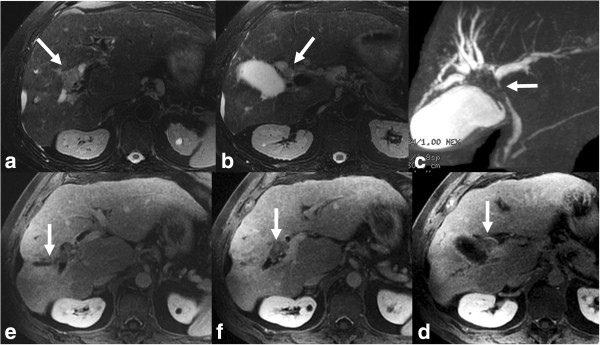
Polypoid type iCCA. Axial T2 sections (a, b) and MRCP (c) demonstrating an isointense filling defect (white arrow) in the right hepatic duct and extending into the common hepatic duct with dilation of intrahepatic ducts. Post contrast enhanced T1-weighted images (d-f) shows the mildly enhancing filling defect representing intraductal papillary neoplasm which extended from just under hepatic capsule filling right hepatic ducts to 3 cm below the confluence of right and left hepatic ducts.
